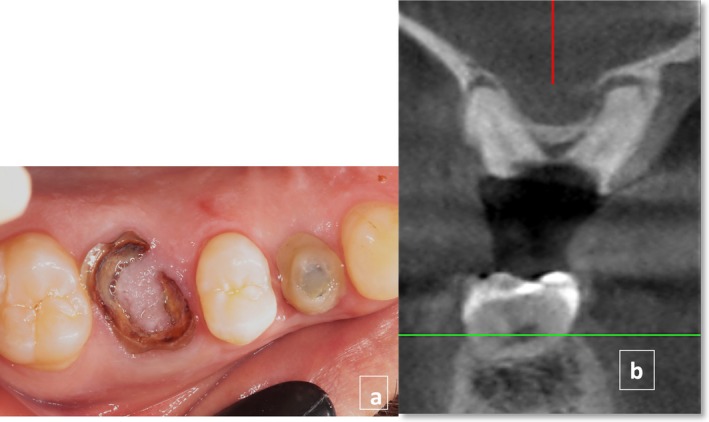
(a) Preoperative clinical view of the decayed maxillary first right molar; (b) CBCT coronal view showing periapical lesions and significant bone defect.
